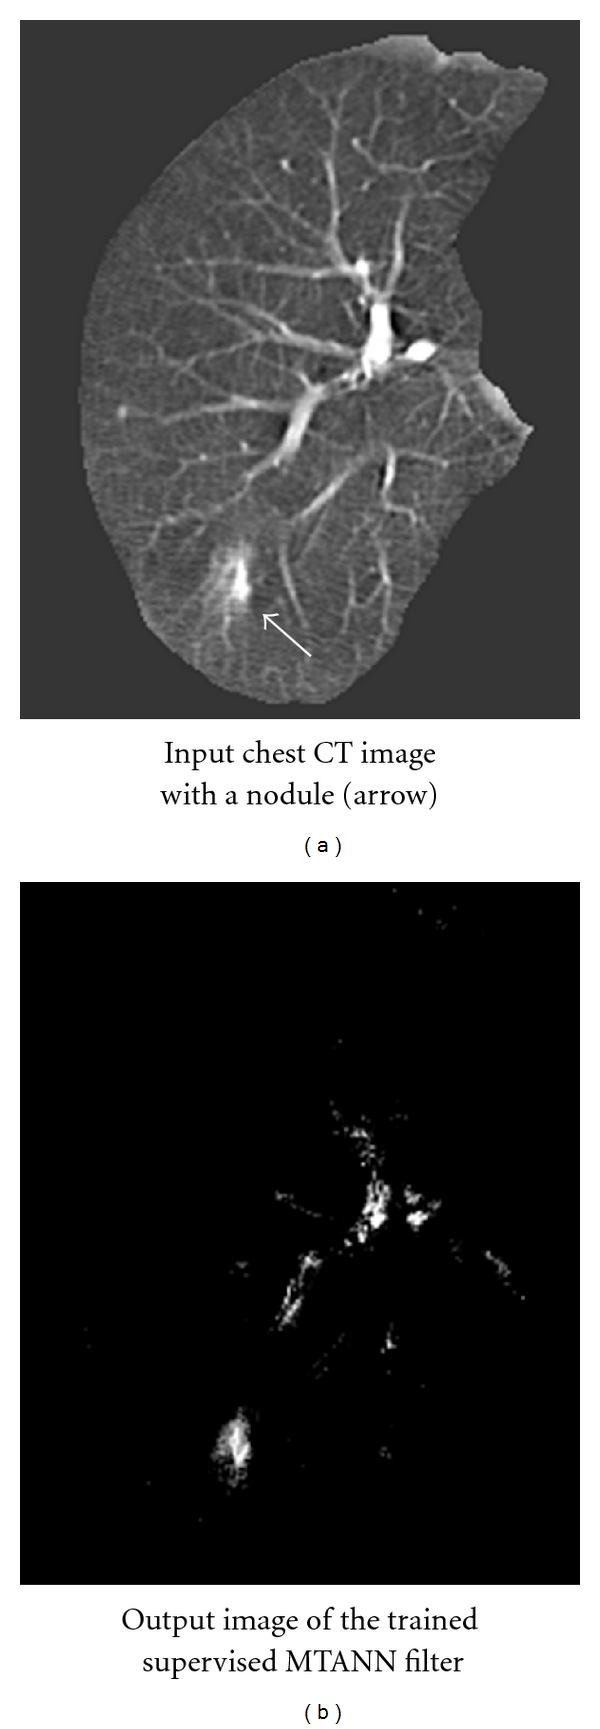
Enhancement of a lesion by use of the trained lesion-enhancement MTANN filter for a nontraining case. (a) Original chest CT image of the segmented lung with a nodule (indicated by an arrow). (b) Output image of the trained lesion-enhancement MTANN filter.
